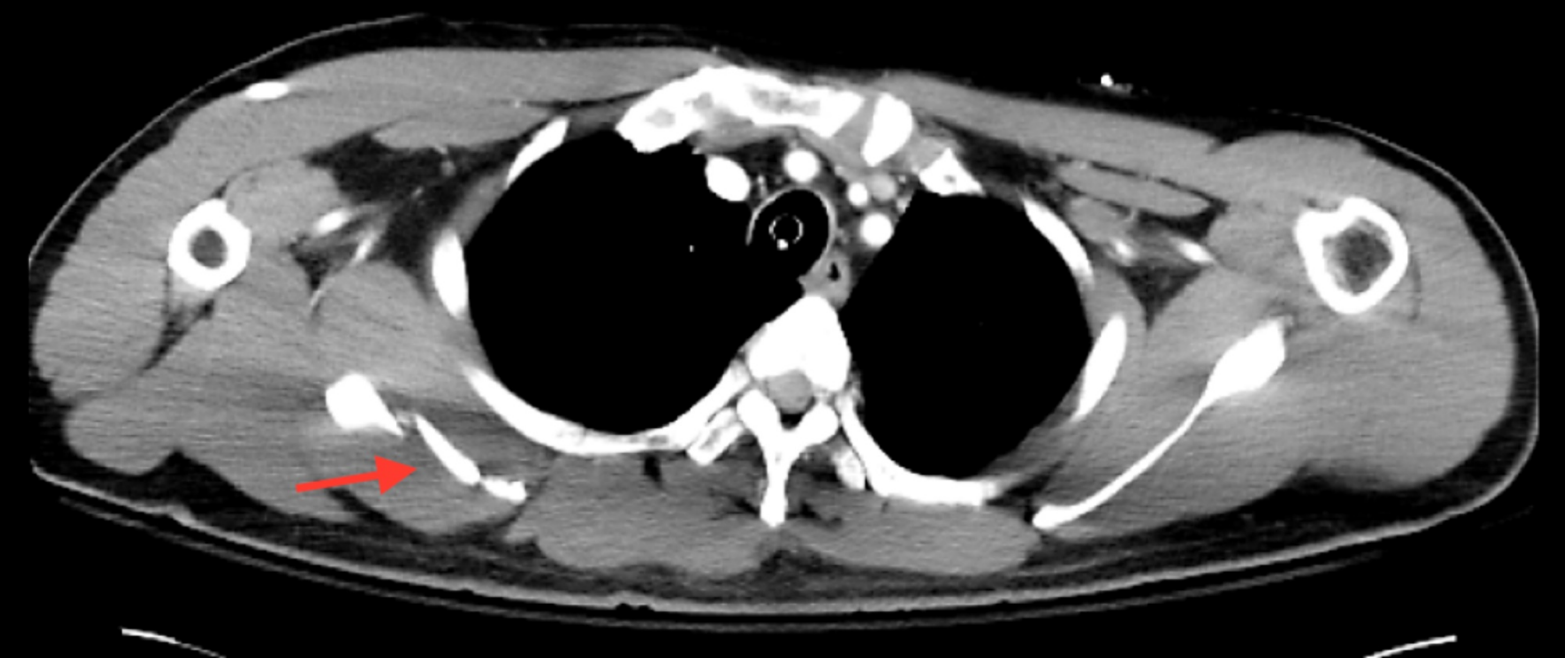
Axial CT demonstrating a right comminuted scapular fracture CT: computed tomography
